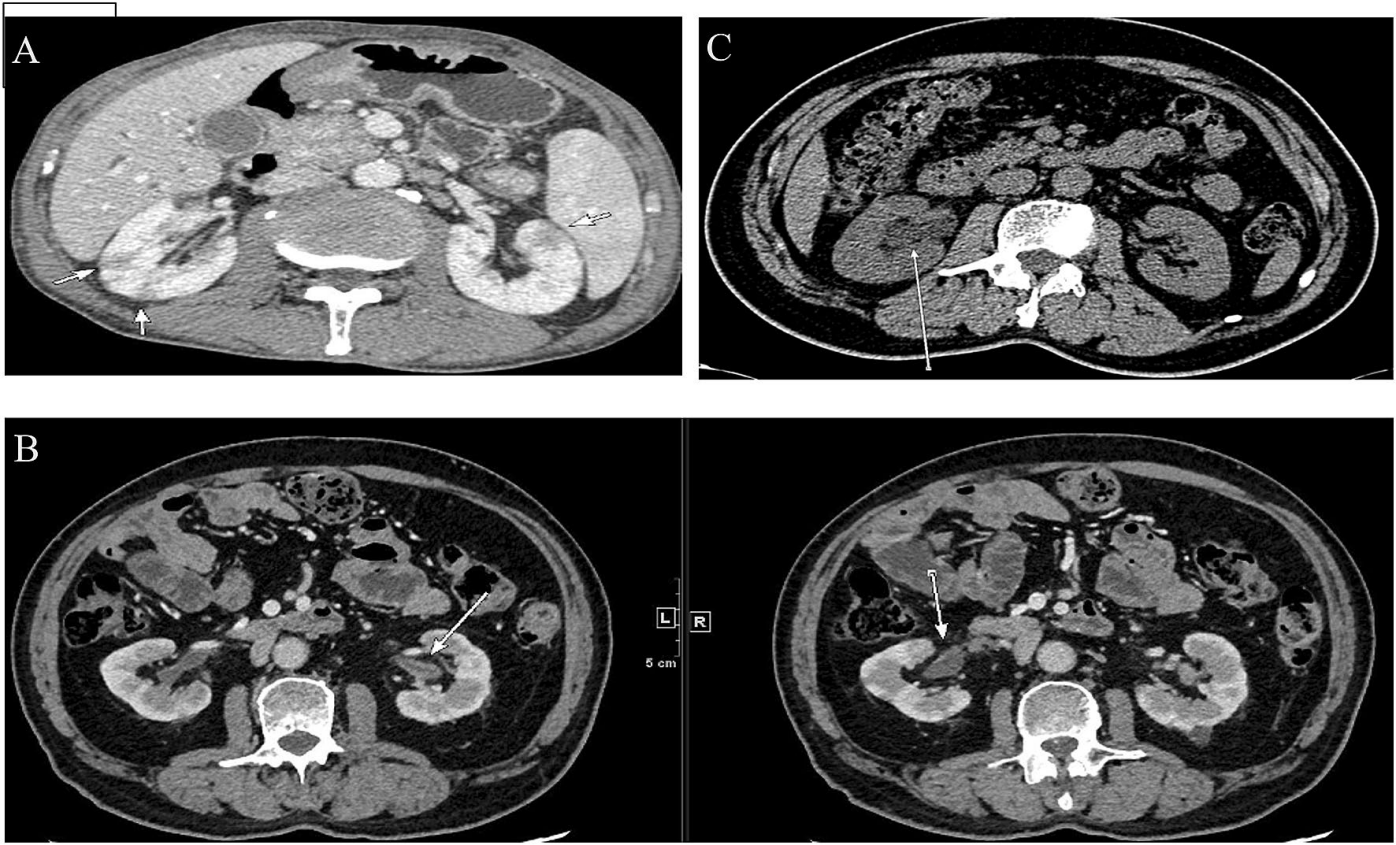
Representative contrast-enhanced CT imaging of IgG4-RKD+ . (A) Multiple low-density lesions in the bilateral kidneys (arrows). (B) ureteric obstruction and hydronephrosis related to RPF (arrow). (C) Renal pelvis thickening with smooth intraluminal surface (arrows).
